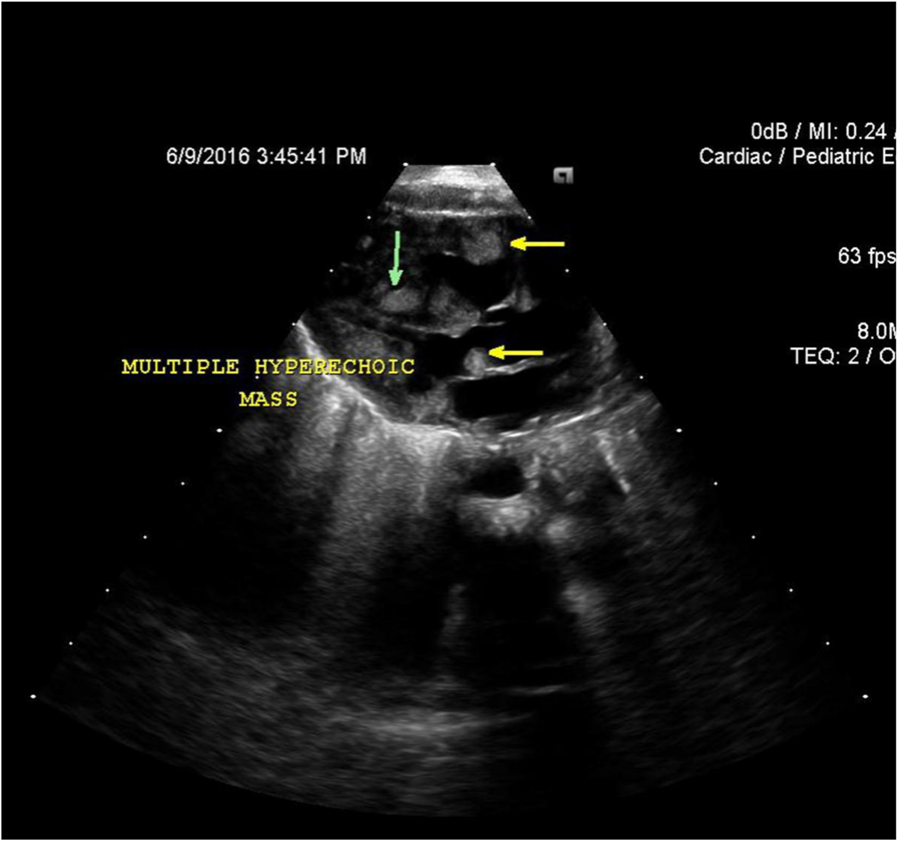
Echocardiogram showing multiple metastatic lesions in the right ventricular free wall (*upper yellow arrow*), aortic valve (*lower yellow arrow*) and right ventricular septum (*green arrow*)
